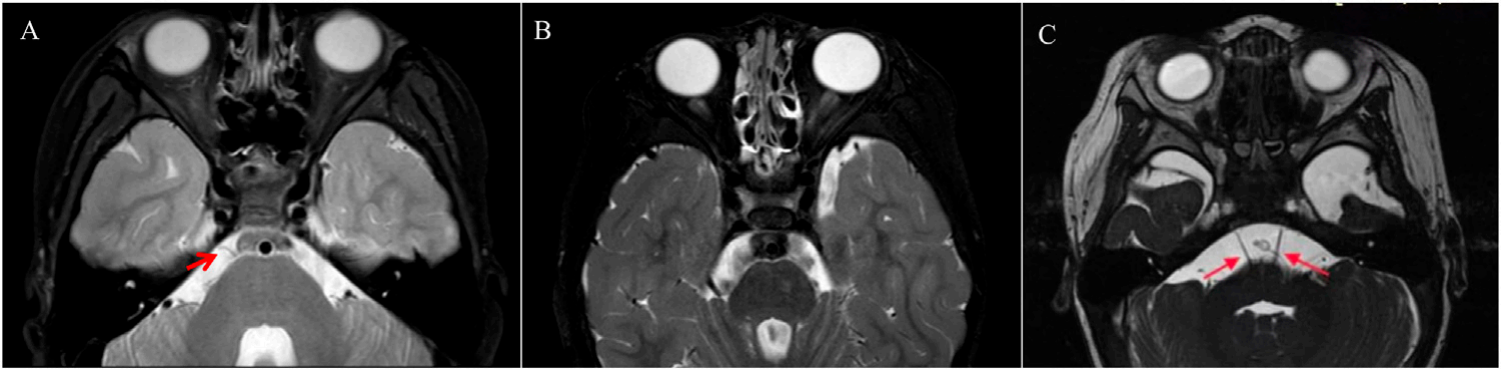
Axial MRI at the pontomedullary junction shows a normal right abducens nerve [(A) arrow]. Both abducens nerves could not be identified in a patient with bilateral DRS (B). Both abducens nerves observed in a patient with DRS2 (C).
